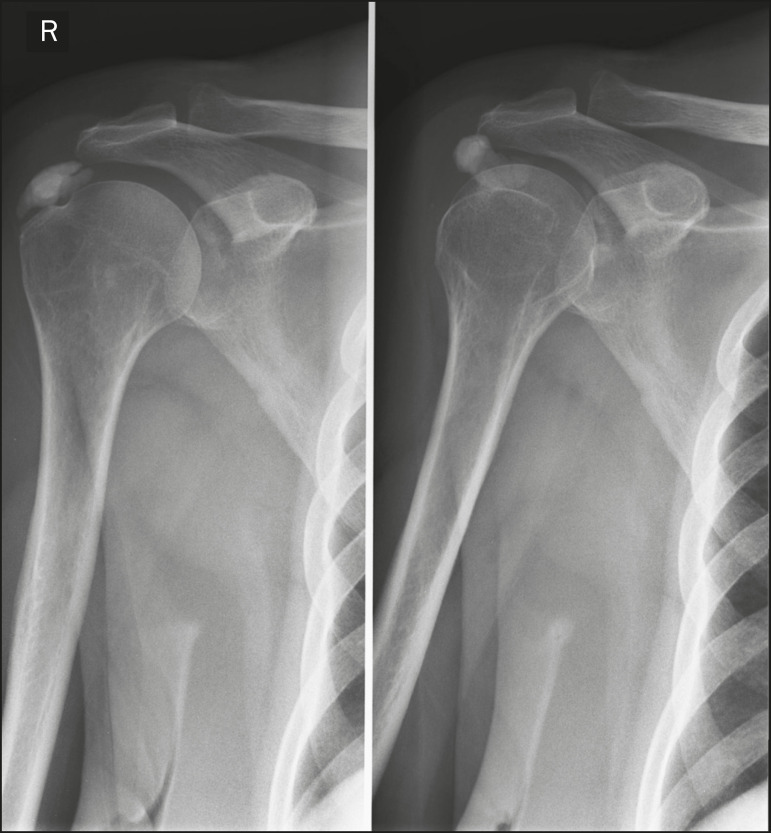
Calcific tendinopathy (hydroxyapatite deposition). Frontal X-ray of the shoulder showing nodular calcifications accumulating in the supraspinatus tendon.
